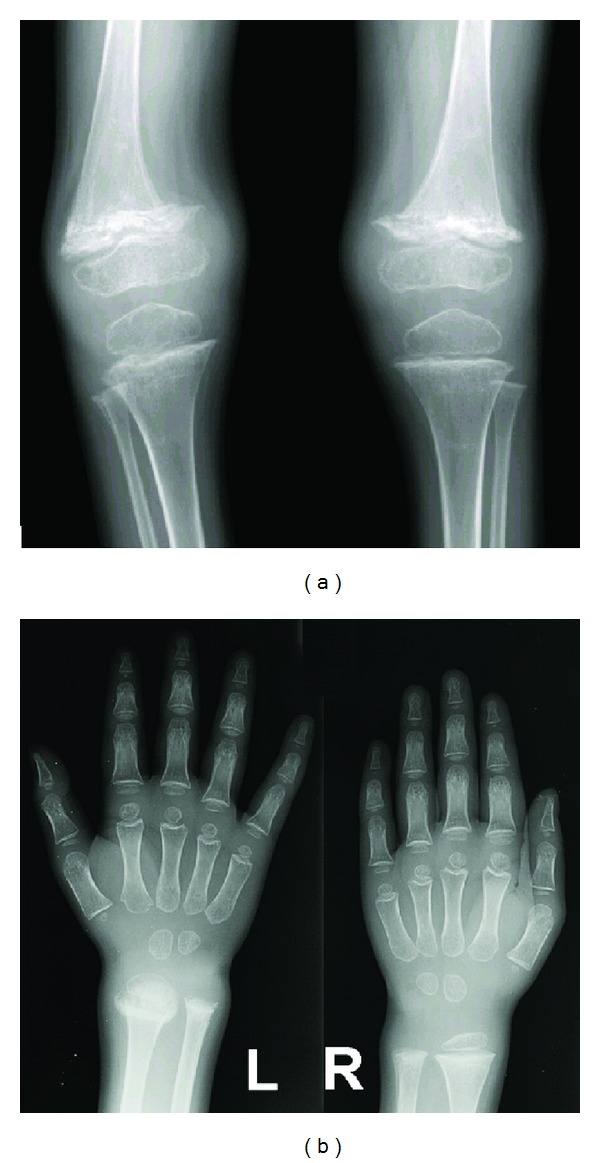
Follow-up X-rays of the knees (a) and wrist (b) at 6 weeks after initiation of vitamin C replacement therapy, showing improvement in osteopenia, healing of the epiphyseal fractures, and reduction in metaphyseal lucencies.
